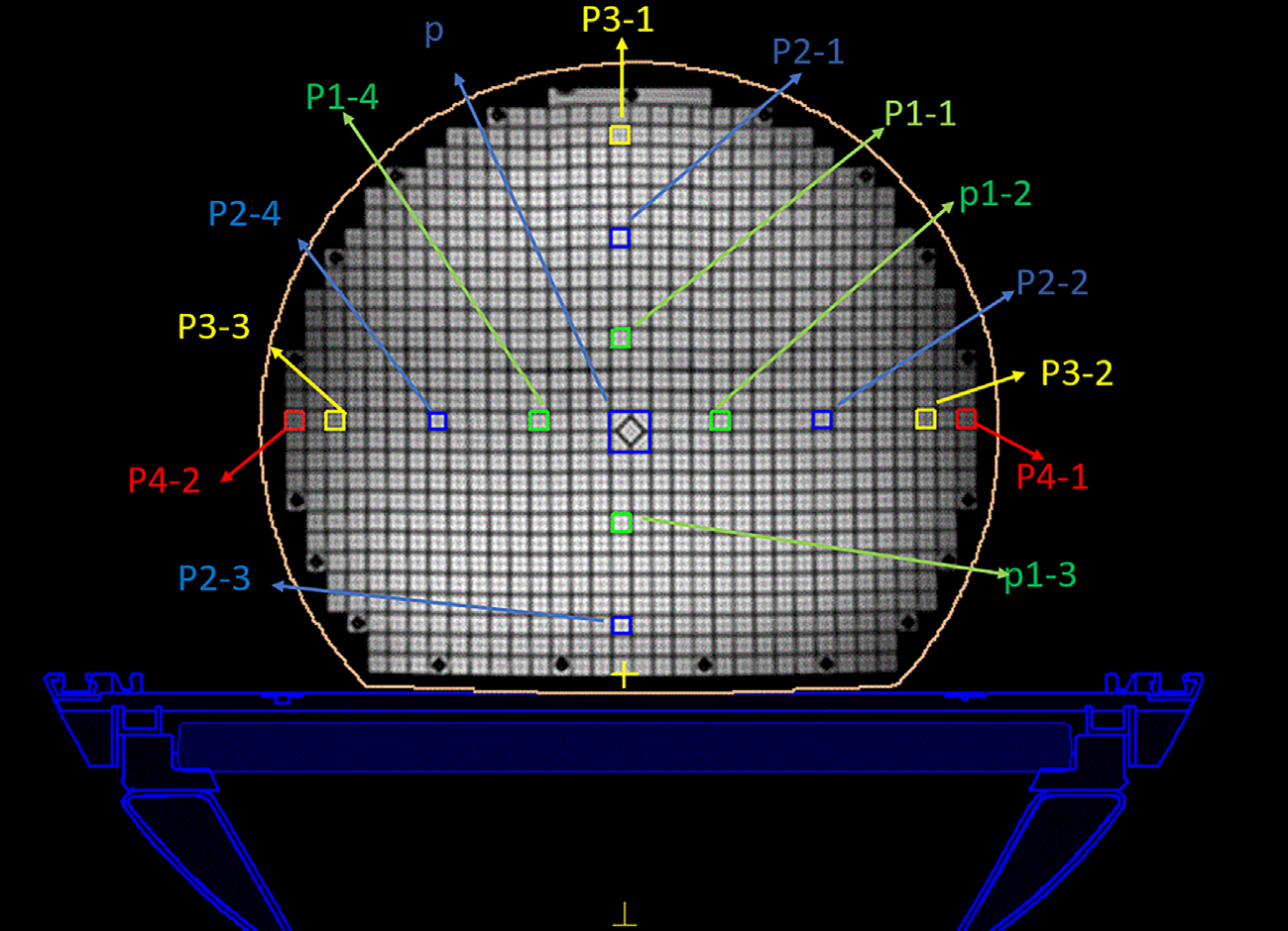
Regions of interest of the MRI image. The center diamond square is regarded as the central point. The green, blue, and yellow circle represent the 5 cm, 10 cm, and 15 cm ranges, respectively.
